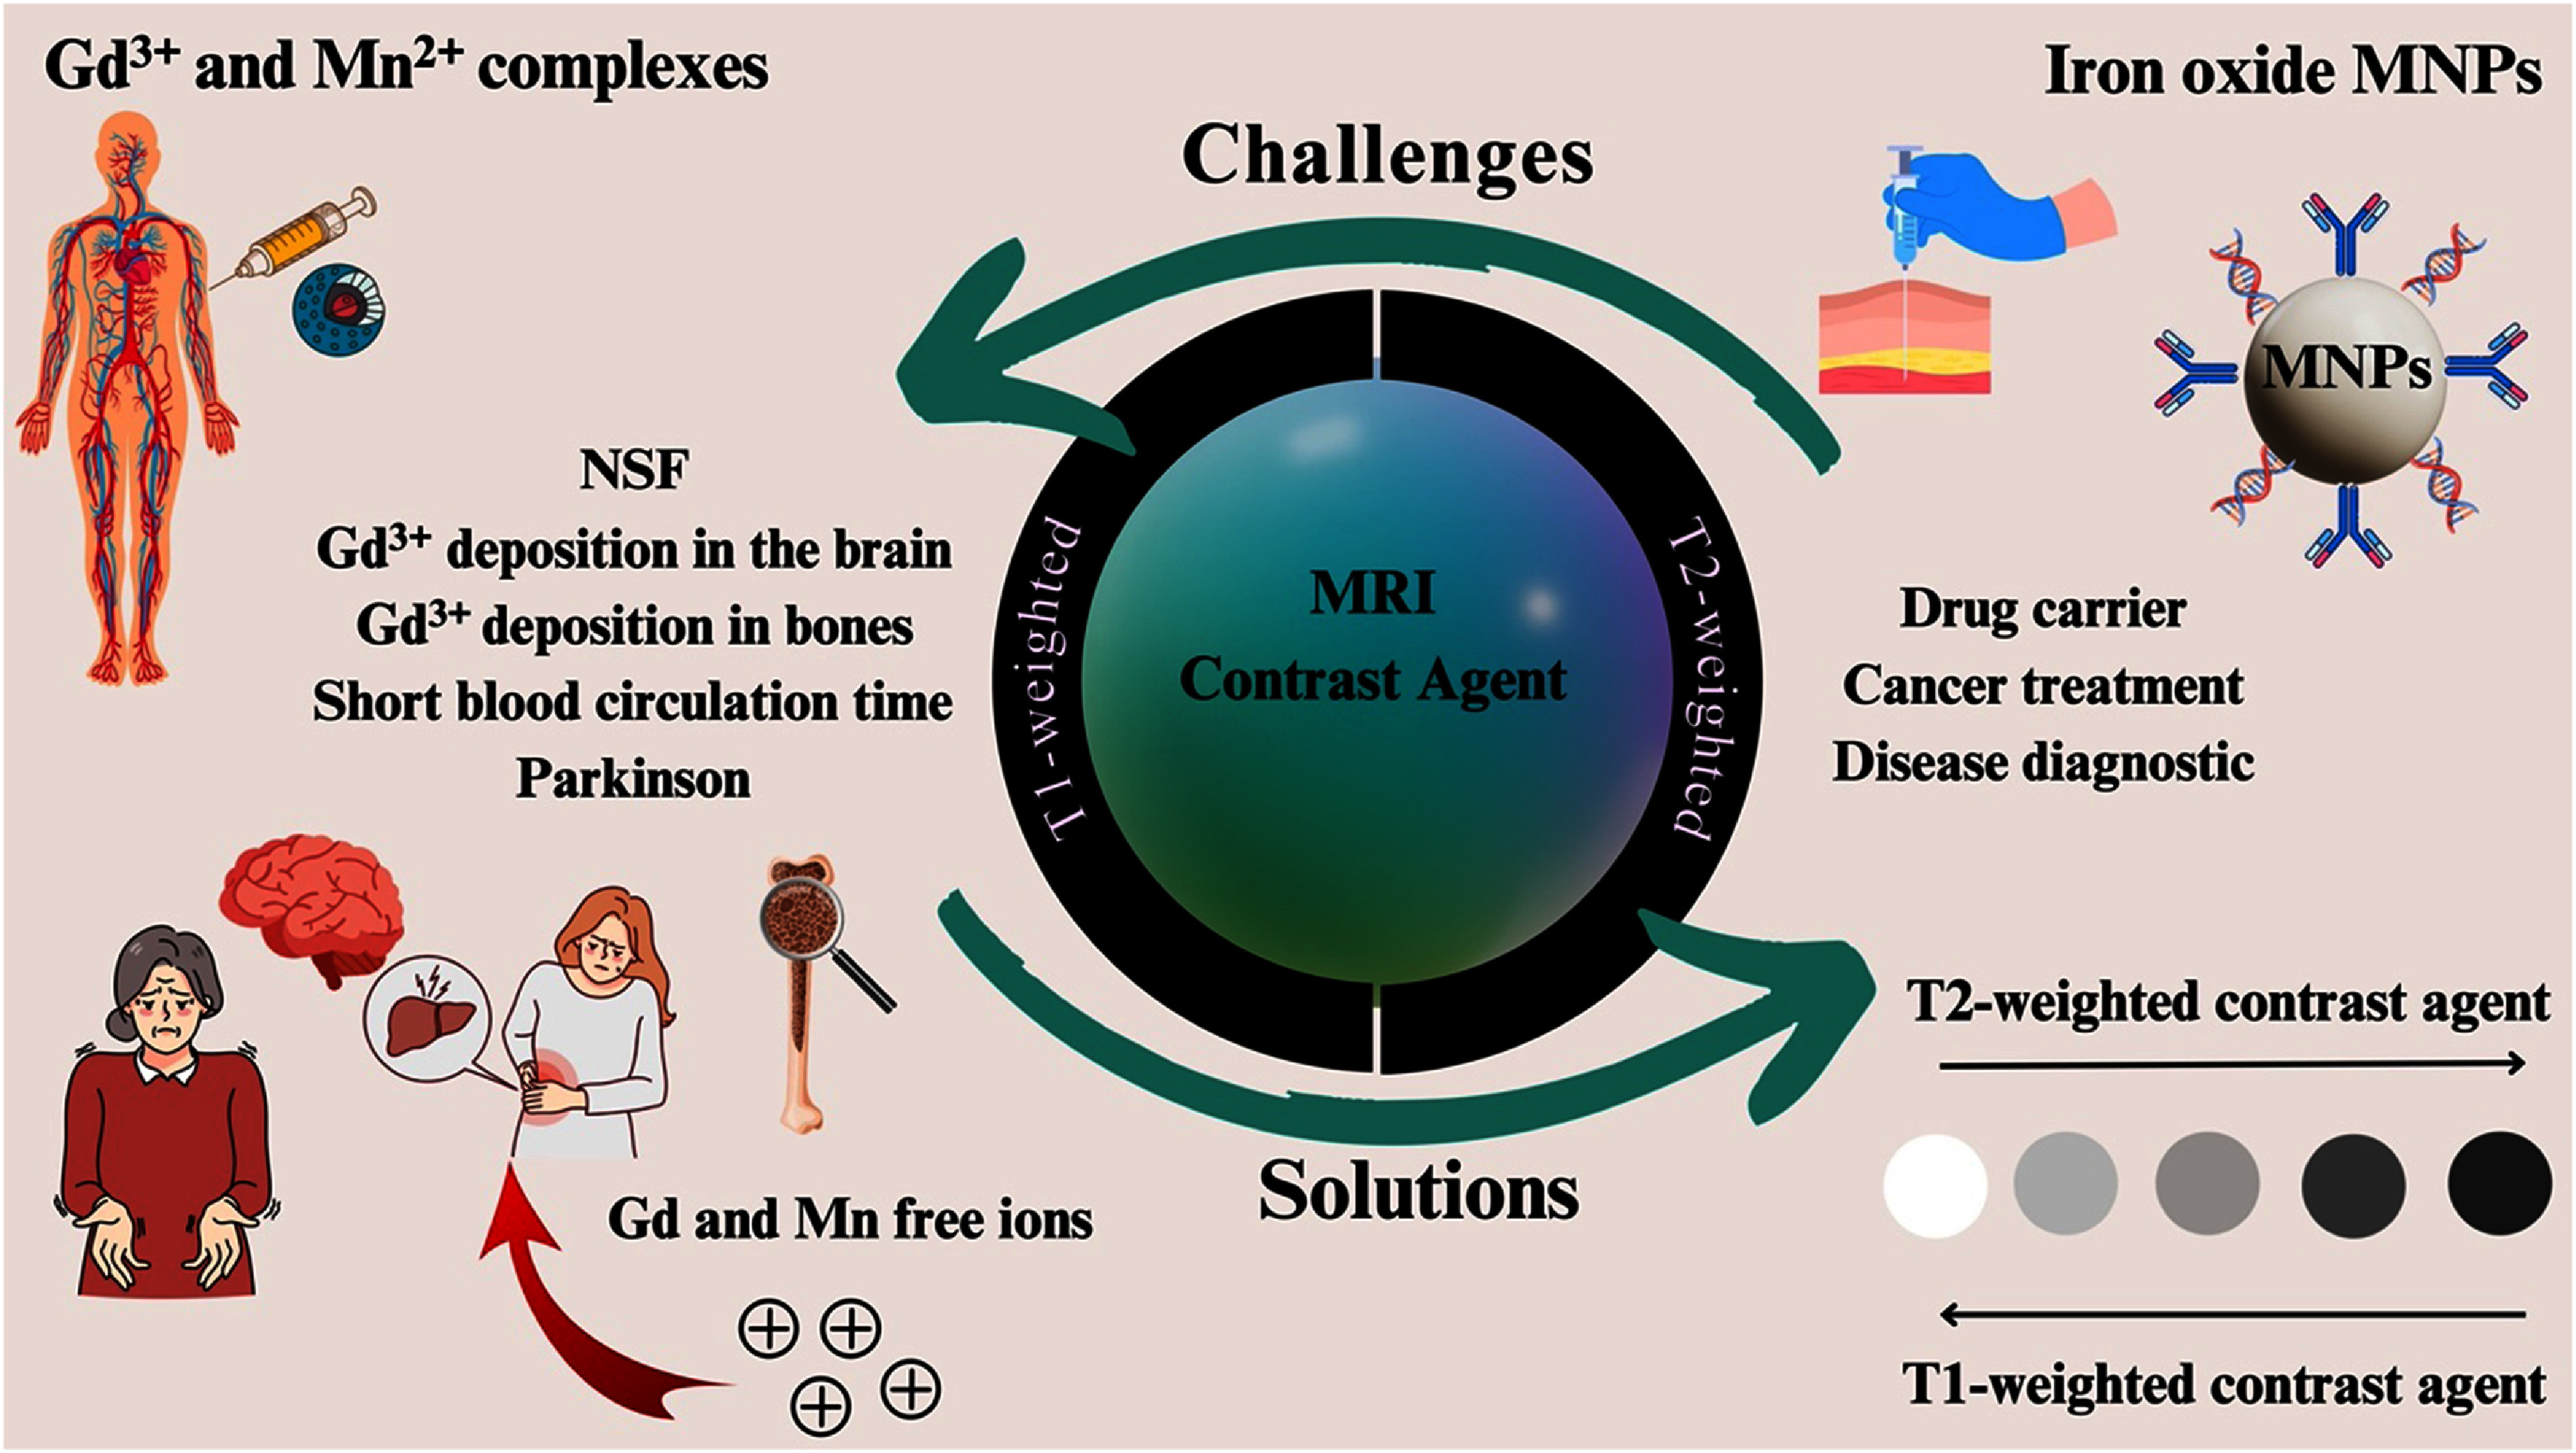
Challenges and opportunities of different MRI contrast agents. Original figure prepared by the authors.
